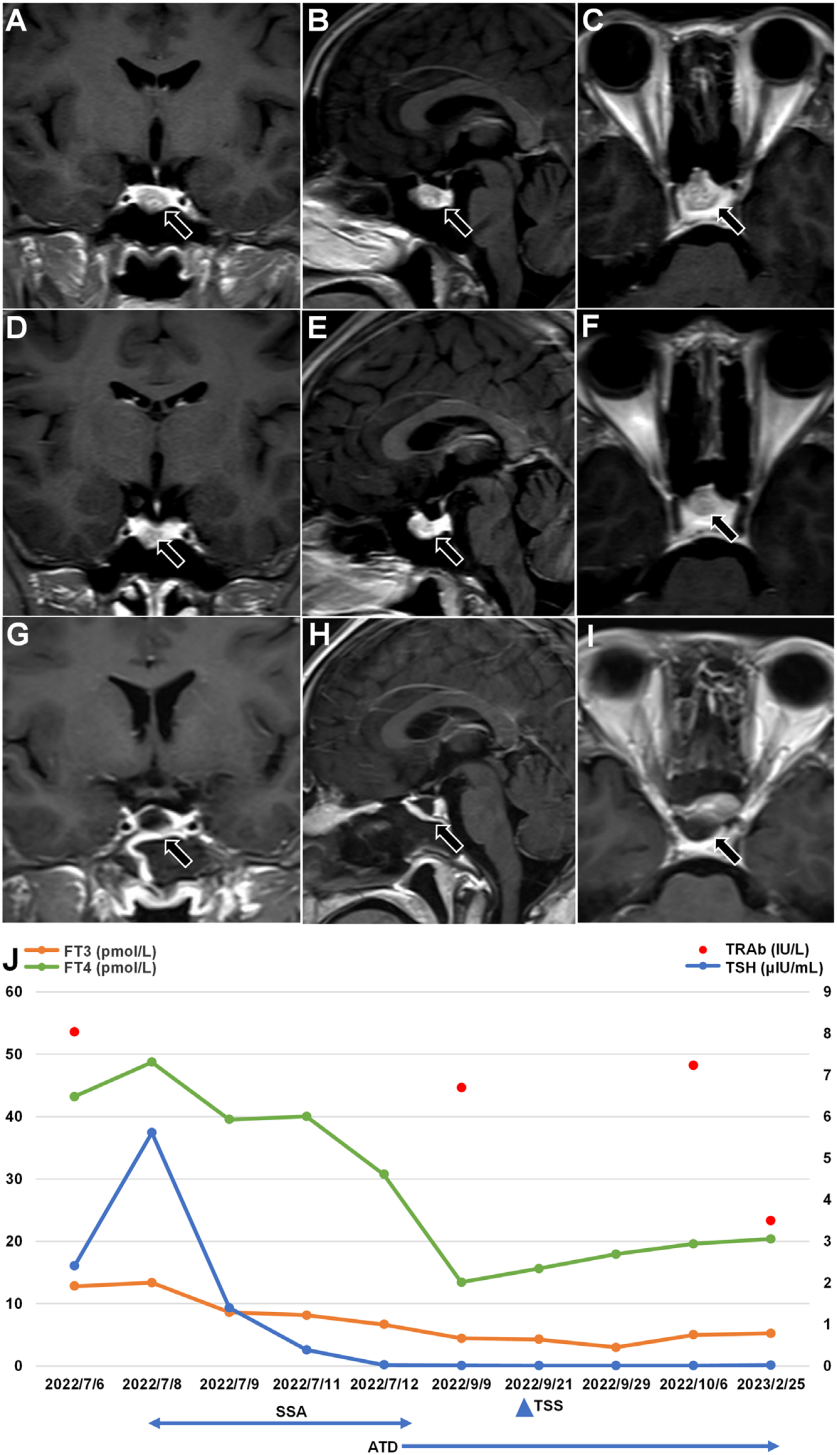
Pituitary enhanced MRI, thyroid function change, and treatment of case 1. ATD, antithyroid drugs; FT3, free tri-iodothyronine; FT4, free thyroxine; SSA, somatostatin analog; TRAb, thyroid-stimulating hormone receptor antibodies; TSH, thyroid-stimulating hormone; TSS, transsphenoidal surgery. Normal ranges: FT4 7.64–16.03 pmol/L, FT3 3.28–6.47 pmol/L, TSH 0.49–4.91 μIU/mL, TRAb 0–1.7 IU/L.
